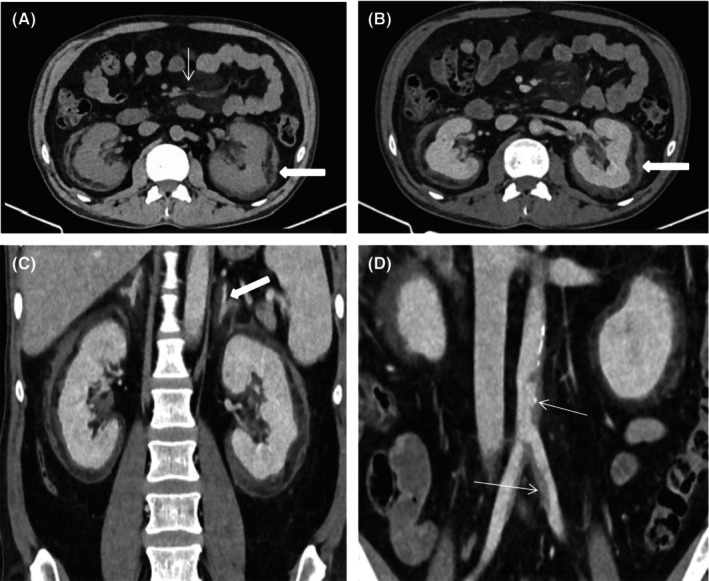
Axial (A and B) contrast‐enhanced CT of abdomen shows ill‐defined, plaque‐like, heterogeneous soft tissue lesion infiltrating bilateral peri‐renal spaces with thickening of anterior and posterior renal fascia (hairy kidney sign; thick arrow) and no sign of hydronephrosis is seen. On the same images, there is fat stranding of the mesentery (thin arrow). Coronal view (C and D) shows mild soft tissue encasement of the abdominal aorta and the proximal bilateral common iliac arteries (Coated aorta sign) (thin arrow) and bilateral adrenal glands (thick arrow).
